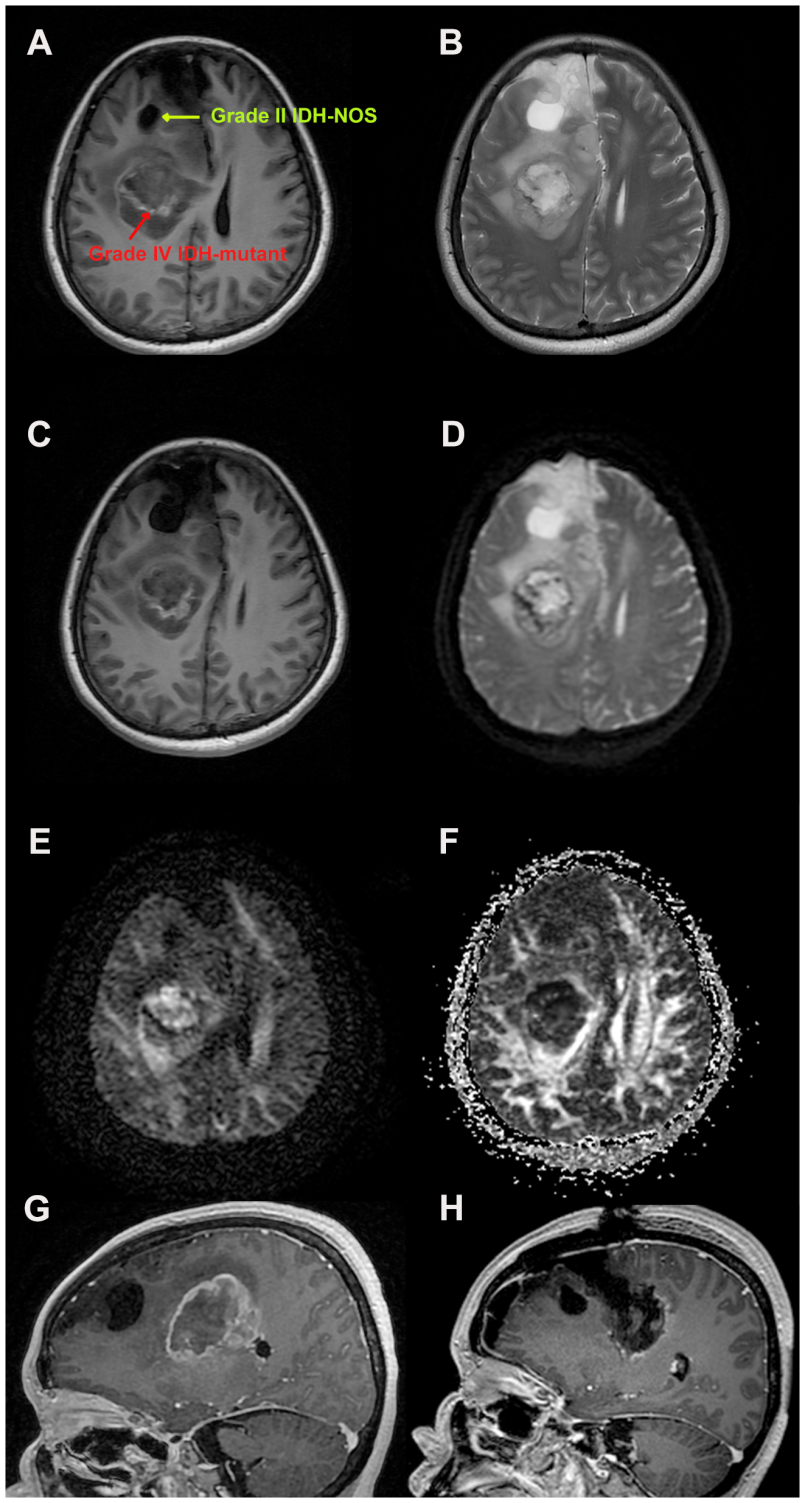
Magnetic resonance imaging findings during the patients’ treatment. (A) Axial post-enhancement T1 weighted images showing a right-sided contrast lesion in the parietal and basal ganglia area, measuring 5.1*4.2*3.7 cm^3^, and a right frontal lobe cystic mass. (B) Axial post-enhancement T2 weighted Propeller images to avoid artifacts. (C) Heterogenous, T1 weighted isometric lesion in the basal ganglia area, with midline shift and sub-calcaneal hernia. (D) Axial T1-FLAIR-weighted images showing parietal lesions as heterogeneous lesions with high contrast frontal cystic masses. Diffusion tensor imaging (E) showing the cerebral neurofibril trajectory and factional anisotropy (F). (G) Preoperative sagittal T1-weighted images reveal two heterogeneous lesions with peripheral edema zone. (H) Postoperative sagittal T1-weighted images showing sub-total tumor resection with partial contrast (to avoid neurological deficits).
